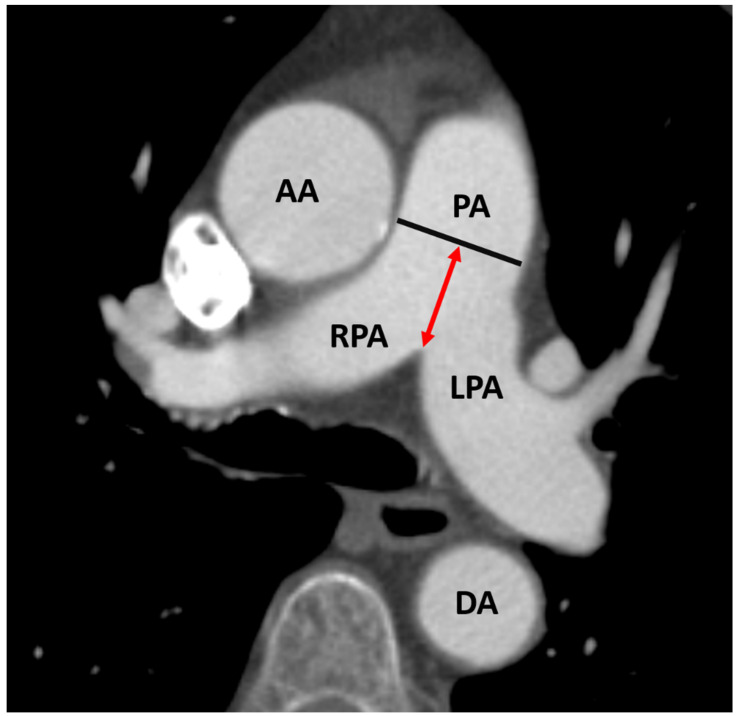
Measurement of pulmonary artery diameter (black line) on axial CT. PA: pulmonary artery; red double-headed arrow: distance between bifurcation of the pulmonary artery and level of measurement within pulmonary artery; RPA: right pulmonary artery; LPA: left pulmonary artery; DA: descending aorta; AA: ascending aorta.
